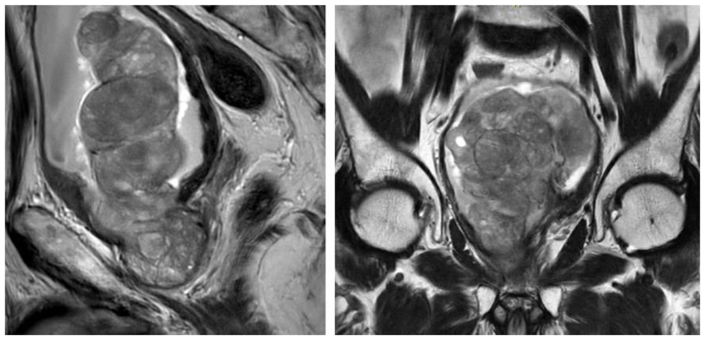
MRI shows intravesical protrusion of giant median prostatic lobe.
